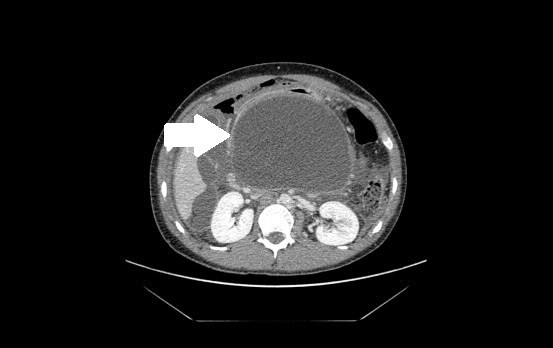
CT scan demonstrating the large pancreatic pseudocyst and free gas within the abdomen. This CT image, taken within a few hours of our patient's admission, shows the large 17 × 11 cm pseudocyst (indicated by the white arrow) and free gas within the abdomen.
